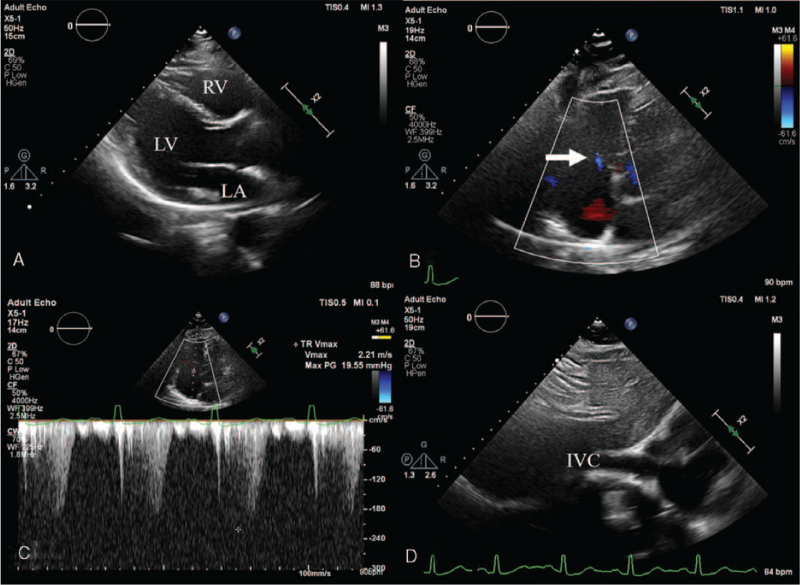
Six-month follow-up echocardiographic images after attainment of euthyroid state showing (A) normalized size of the right ventricle, (B) near complete resolution of tricuspid regurgitation (arrow), and (C–D) normalization of pulmonary artery systolic pressure and inferior vena cava diameter. IVC = inferior vena cava, LA = left atrium, LV = left ventricle, RV = right ventricle.
